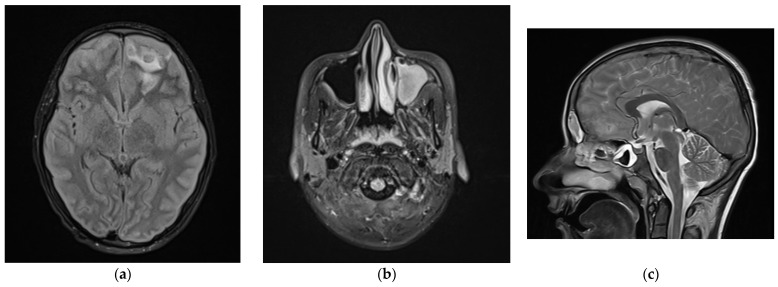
Magnetic resonance imaging (MRI) of the head of 10 December 2021 revealed: (a) in the left frontal lobe several irregular fluid areas features of intracerebral abscesses with mass effect; (b) massive inflammation of the left maxillary sinus; (c) sagittal sinus thrombosis.
